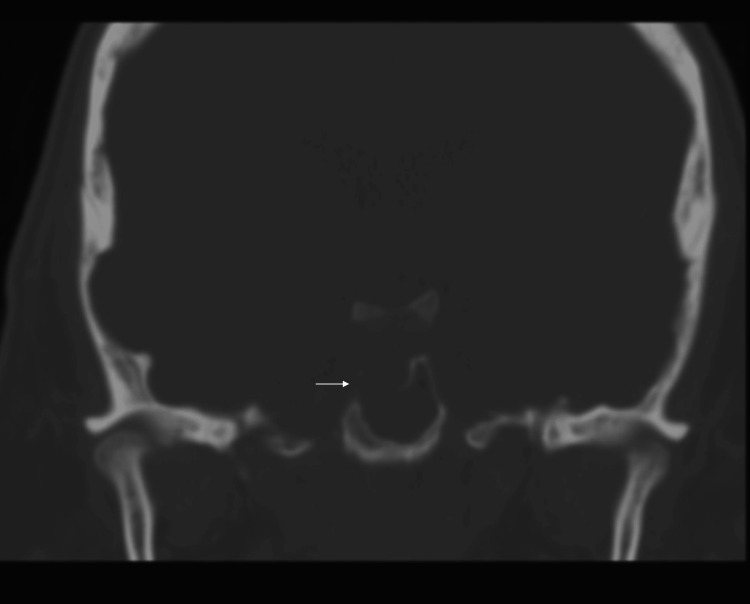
CT sinus showing apparent destruction of the right sellar floor
